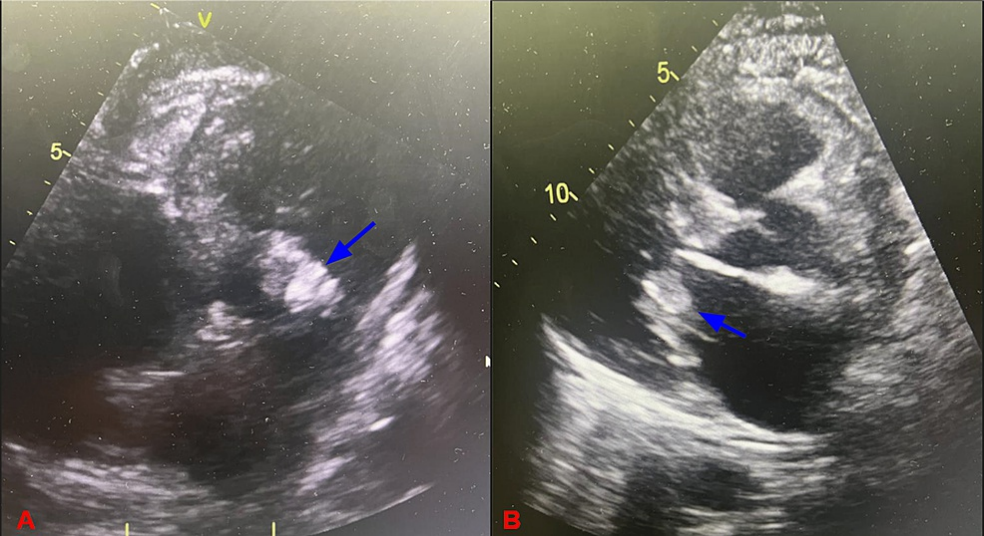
Echocardiographic image showing left-sided pleural effusion and mobile vegetation (blue arrows) on the posterior mitral leaflet, measuring 3.0 x 1.9 cm.
